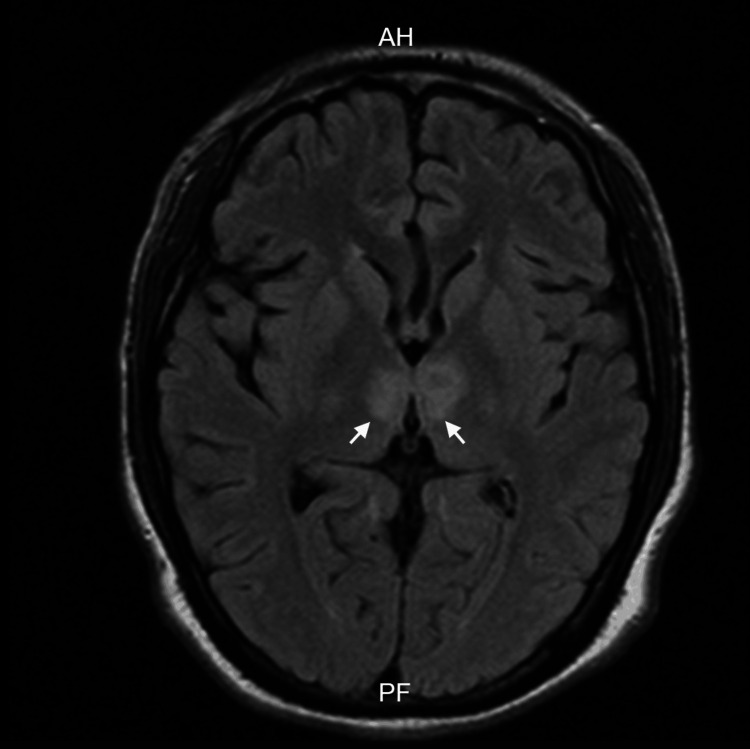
MRI FLAIR of bilateral thalamic infarction secondary to occlusion of the artery of Percheron Axial fluid-attenuated inversion recovery (FLAIR) demonstrates a bilateral hyperintense lesion located within both thalami. AH: Acute hemorrhage; PF: Posterior fossa.
